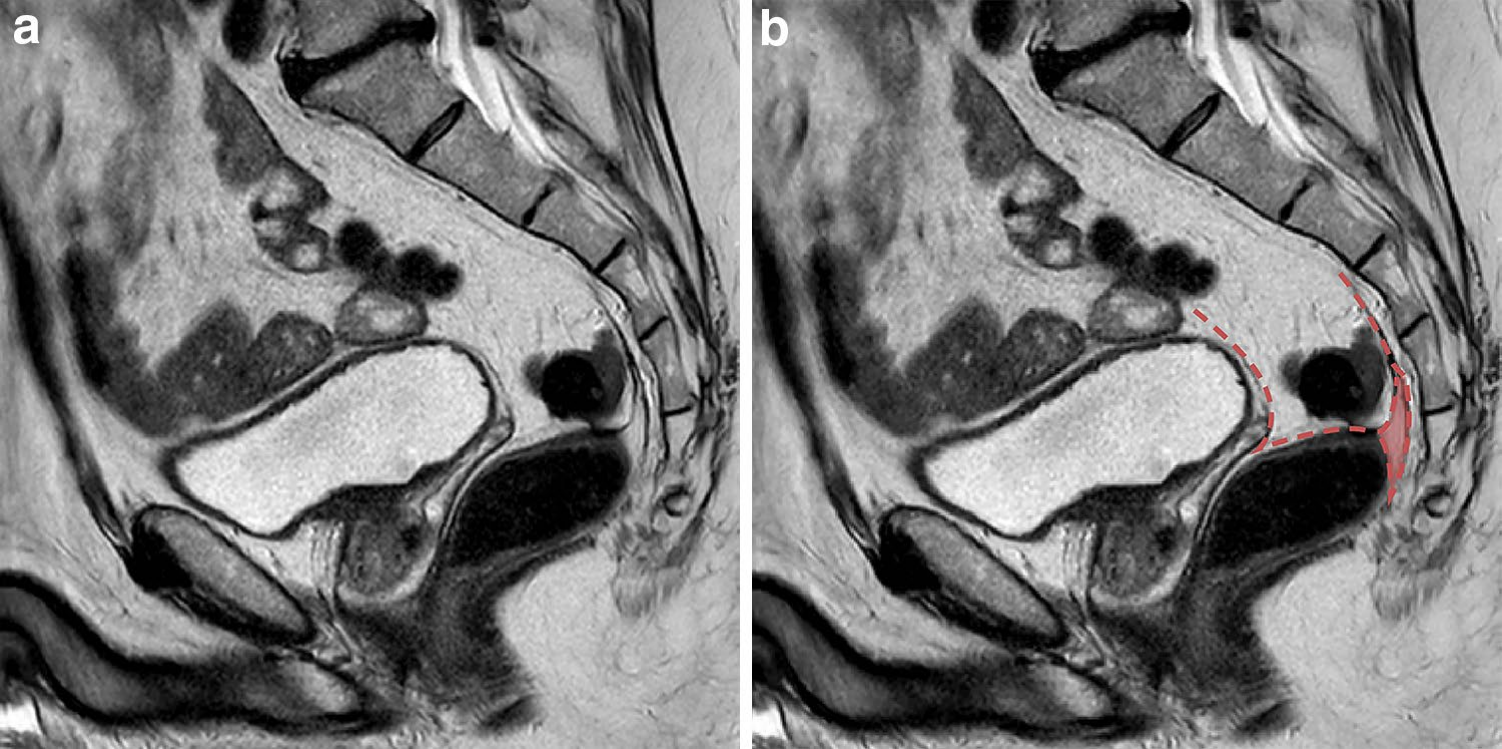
MRI-detected residual mesorectum following laparoscopic total mesorectal excision (LapTME). a, b Sagittal T2-weighted MR-images showing residual mesorectum below the anastomosis and perianastomotic. b Residual mesorectum coloured in red. Red dashed line is showing the level of dissection and anastomosis after total mesorectal excision. (Color figure online)
